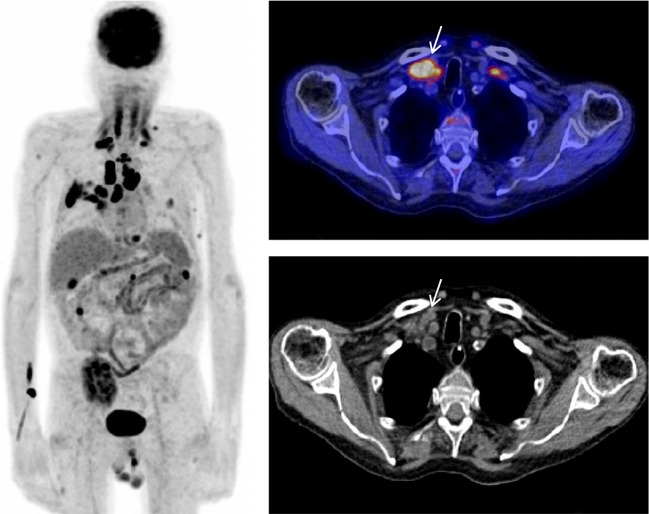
A 62-year-old male in which elevated LDH levels (347 U/l) and EBV-DNA load (1,032,500 copies/ml) were found after clinical monitoring within 1 year after kidney transplantation. FDG-PET/CT was subsequently requested. Maximum intensity projection FDG-PET shows disseminated metabolically active cervical, mediastinal, and lung parenchymal lesions with focal pararenal, native kidney, mesenteric, and liver lesions. Axial fused FDG-PET/CT (top right) and CT (bottom right) show a metabolically active supraclavicular lymph node that proved to be a granulomatous inflammation due to a mycobacterium after biopsy
